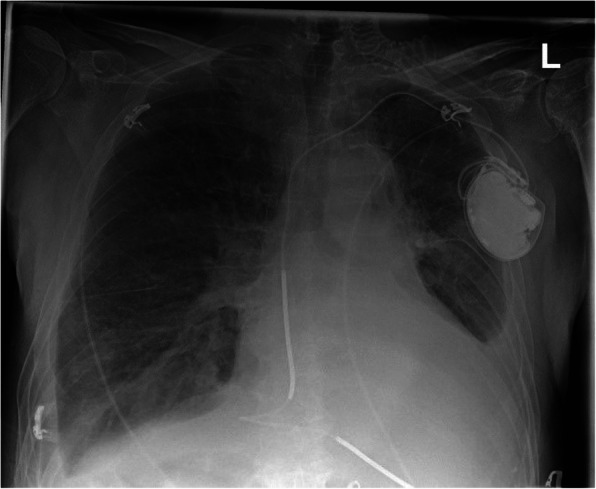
X-Ray 03/25/2020, left-sided pulmonal fluids
